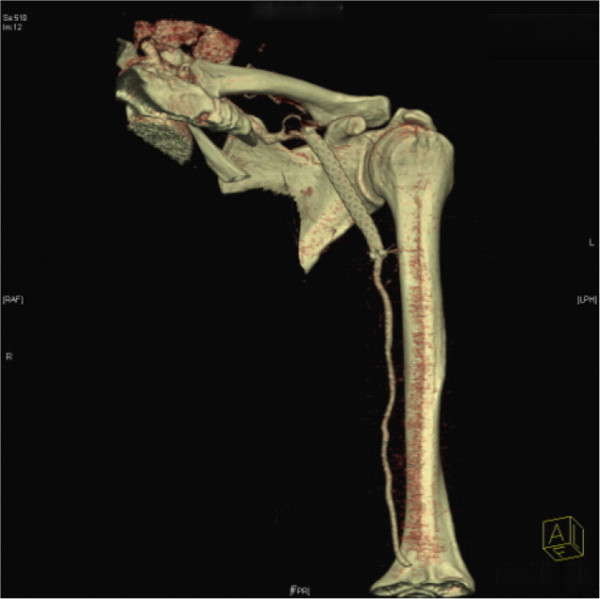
Computed tomography (CT) angiography showed complete exclusion of the aneurysm without any endoleaks.
